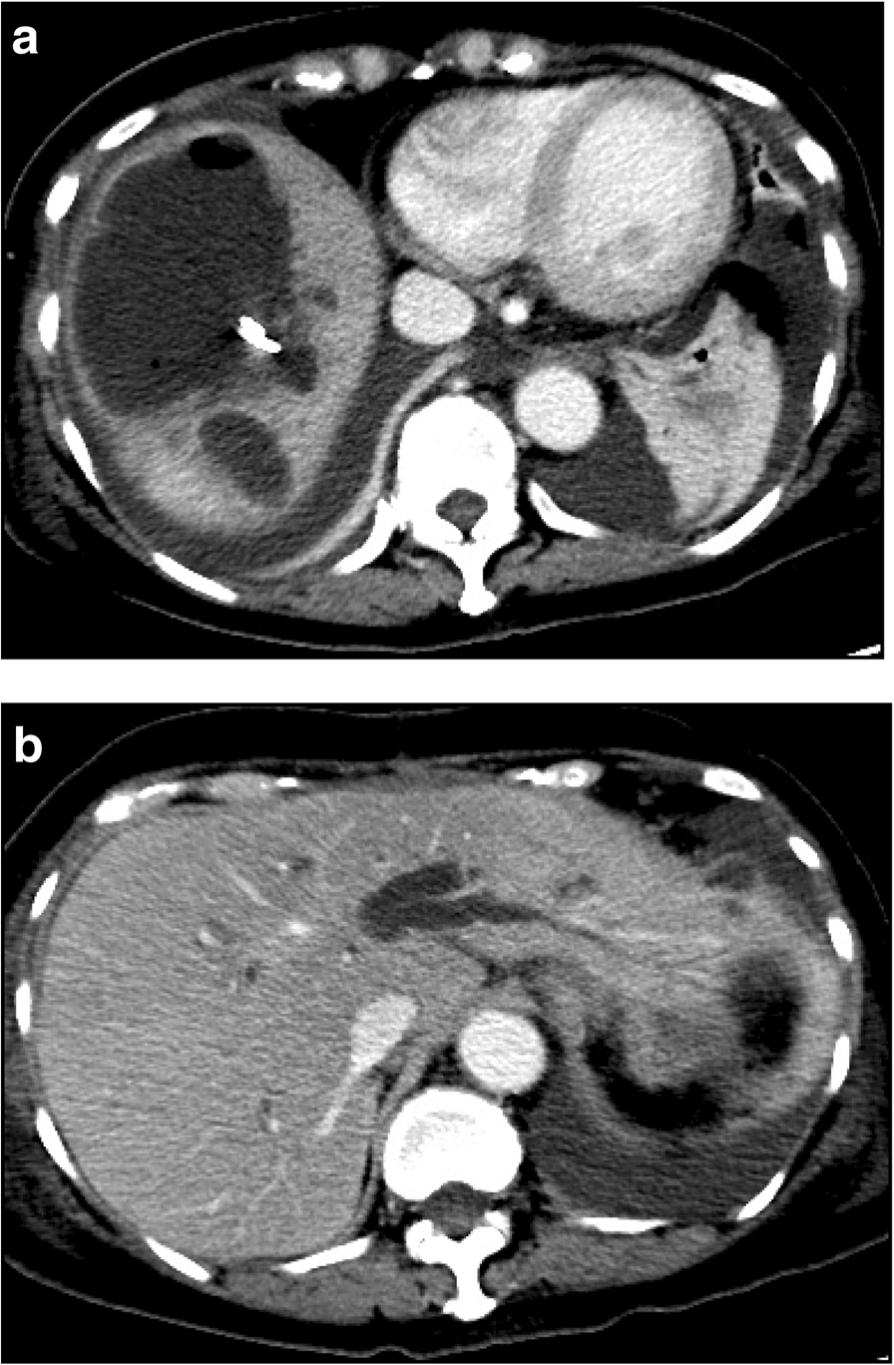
Contrast-enhanced multidetector CT at POD 65 showing severe hepatic cytolysis (a) and choledochojejunostomy stenosis (b)
